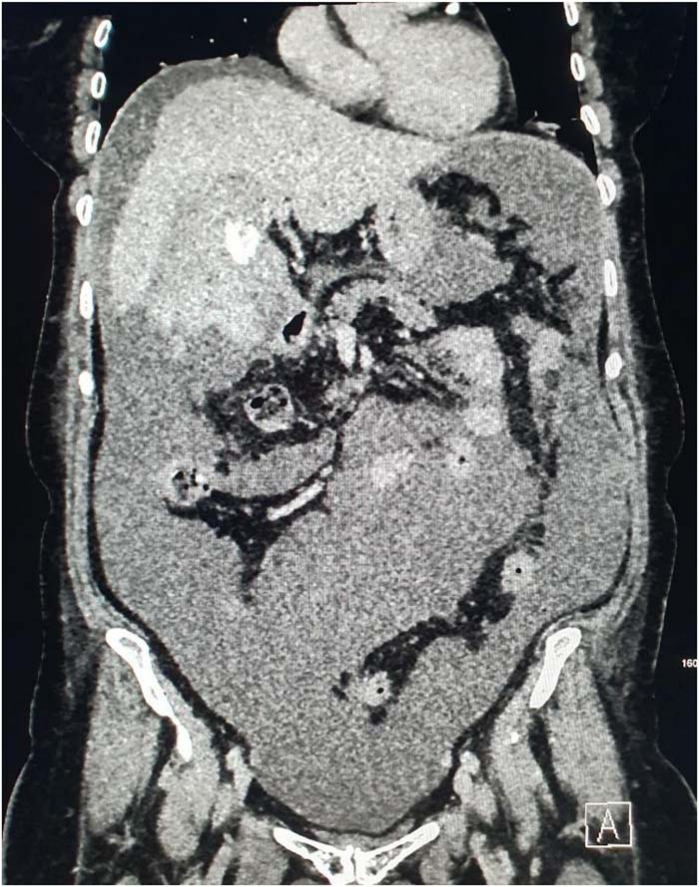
CT demonstrating large volume of bilious peritoneal fluid with haematoma and calculus in the gallbladder fossa.
